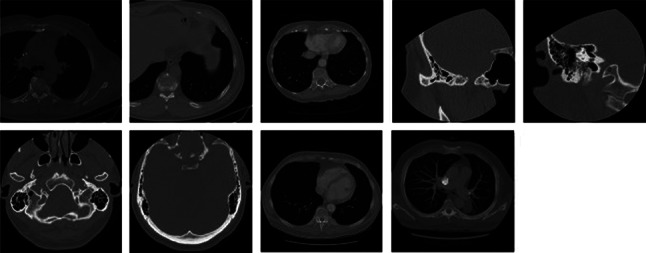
Sample images from each class of NEMA-CT database
